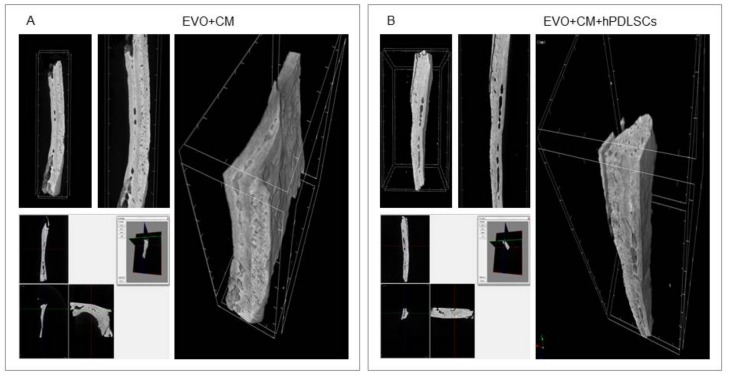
Micro-computed tomography (CT) analyses. 3D volume rendering and 2D virtual sectioning in the 3 orthogonal planes of EVO + CM (A) and EVO + CM + hPDLSCs (B).
